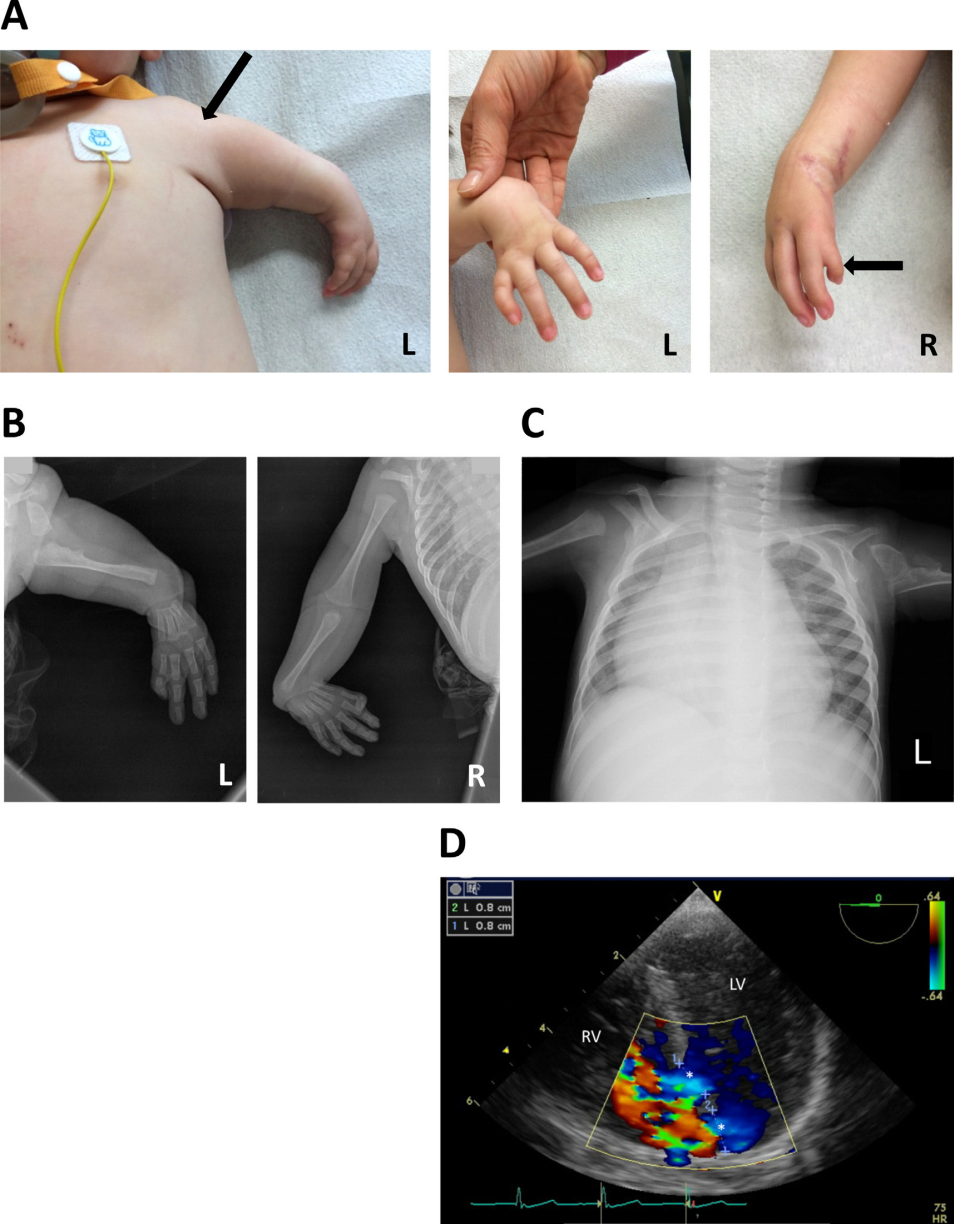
Upper limb anomalies and cardiac defects of the patient. (A) Photographs of the body and upper limbs showing the deformation of the upper arms and thumbs on both hands. (B) X‐rays of both upper limbs. (C) X‐ray of the body. (D) Echocardiogram showing the two VSDs (marked by *).
